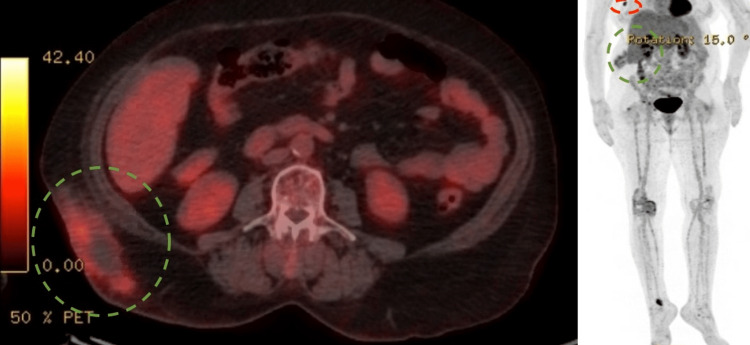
PET-CT scan showing mild heterogeneous soft tissue nodularity with central photopenia and diffuse FDG-avid activity seen in the vicinity of resected right lumbar subcutaneous lesion (green dashed circle) and mild FDG-avid right axillary lesion (red dashed circle)
